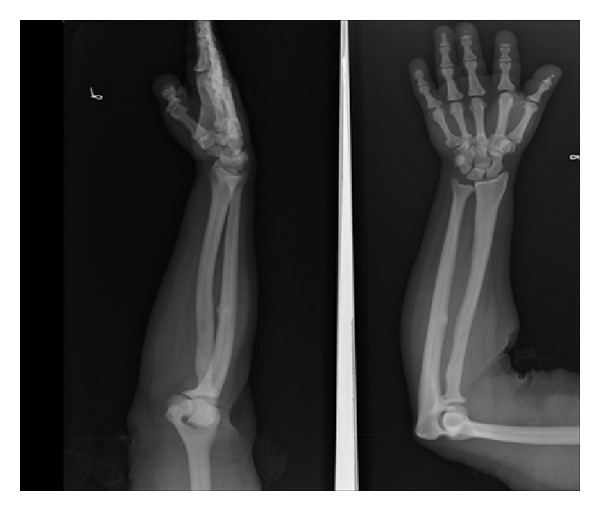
X-ray showing sclerosis of terminal phalanges.
